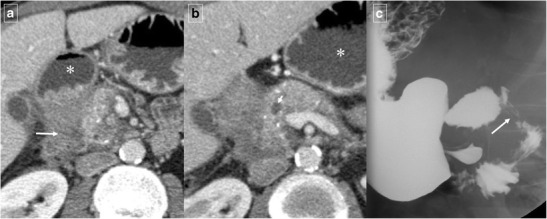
A 55-year-old man with history of alcohol abuse and chronic pancreatitis. Axial contrast-enhanced CT images (a and b) demonstrate atrophy of the pancreatic parenchyma and ductal dilation (short arrow). A stricture of the duodenal lumen (arrow) caused by fibrotic changes in the pancreaticoduodenal groove is accompanied by gastric dilation (*). c Image from a barium study shows a stricture in the proximal duodenum with an abnormal mucosal pattern (arrow)
